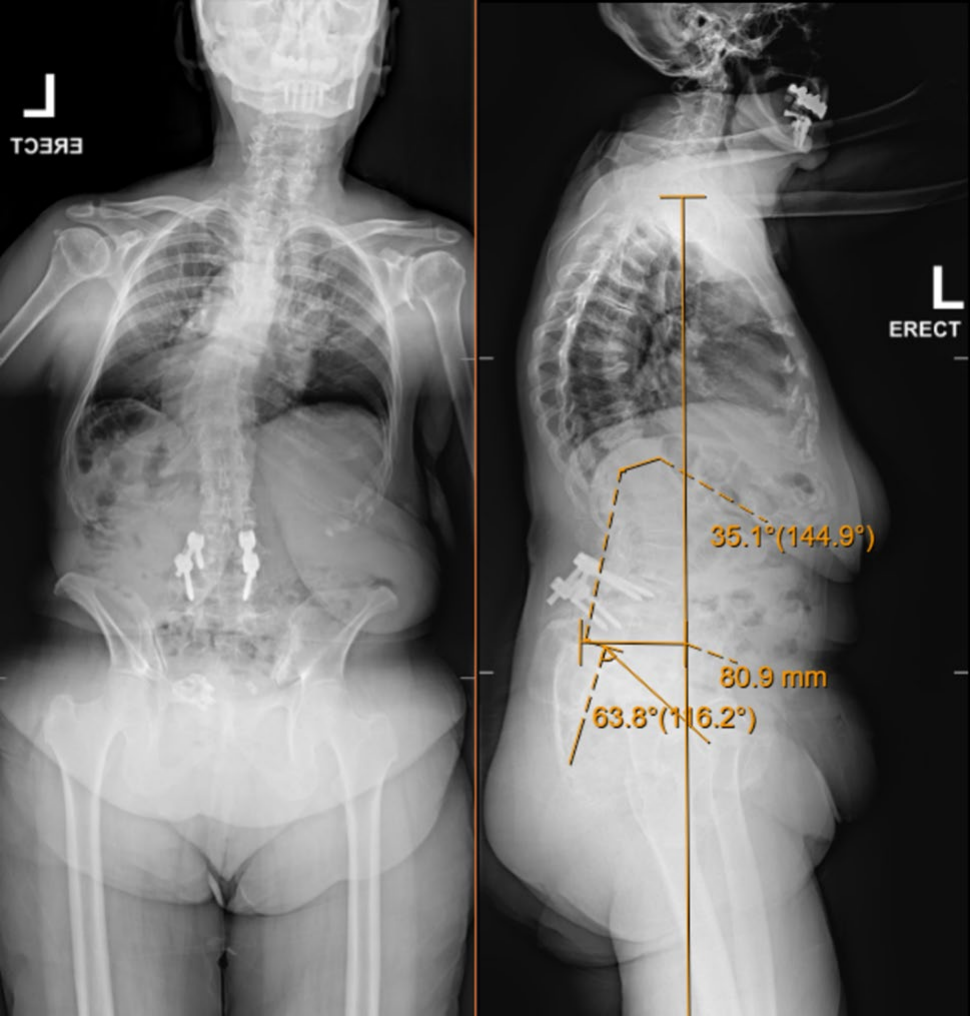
74-year-old patient with a history of L4-5 posterolateral fusion presenting with left-sided low back pain, difficulty with upright posture, and progressive lower extremity paresthesias and weakness secondary to spinal stenosis. Preoperative X-rays (PA and lateral) of the thoracolumbar spine show sagittal imbalance with SVA 8.9 cm and Pelvic incidence—Lumbar Lordosis mismatch of ~ 28 degrees. Retained implants in segmental kyphosis at L4-L5 and previous L5-S1 fusion
